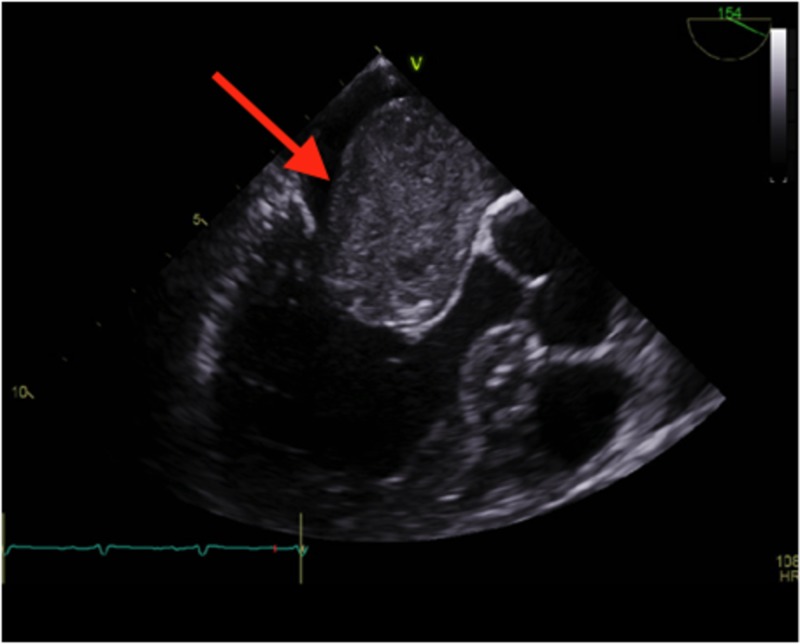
Transesophageal echocardiogram Red arrow showing left atrial mass
